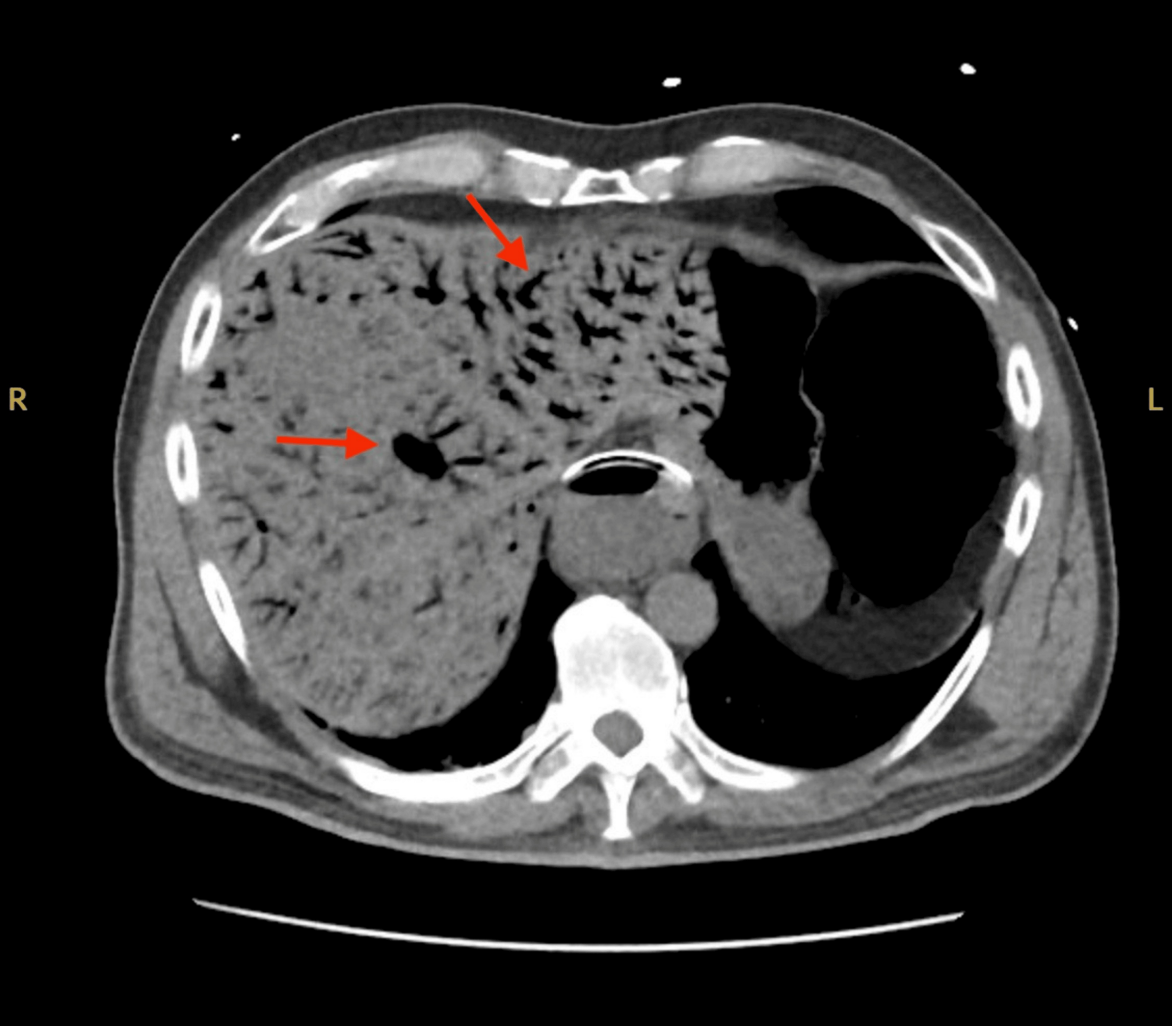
Axial CT scan of the abdomen and pelvis without contrast showing pneumatosis in the liver and portal venous gas
